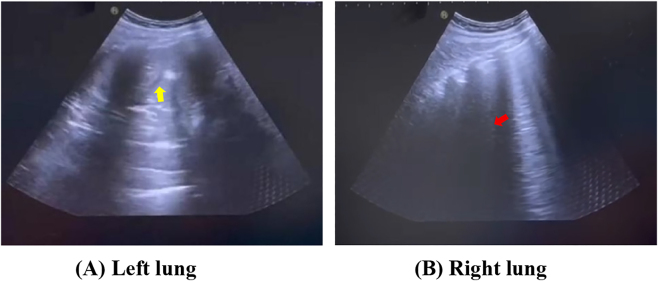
Bedside lung ultrasound performed immediately after surgery. (A) Left lung consolidation (yellow arrow). (B) Numerous B-lines in the right lung (red arrow).
